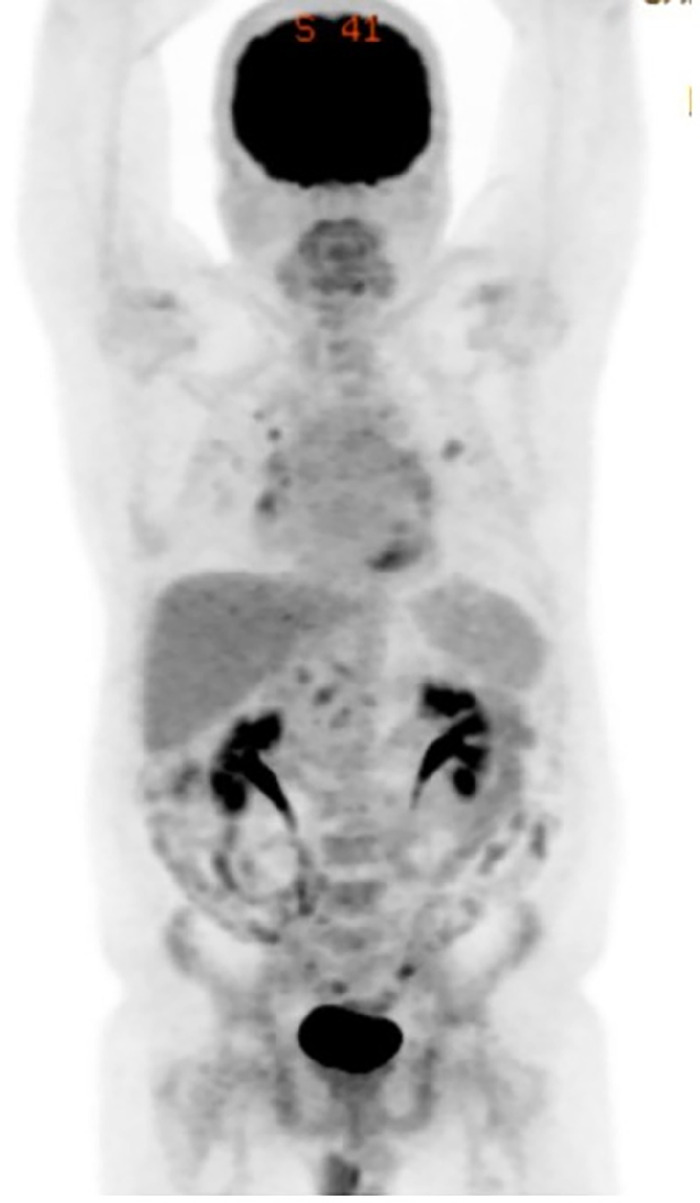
Maximum intensity projection positron emission tomography-computed tomography imaging obtained nearly a year postoperatively shows no esophageal mass but does show some reactive hilar and mediastinal lymph nodes.
